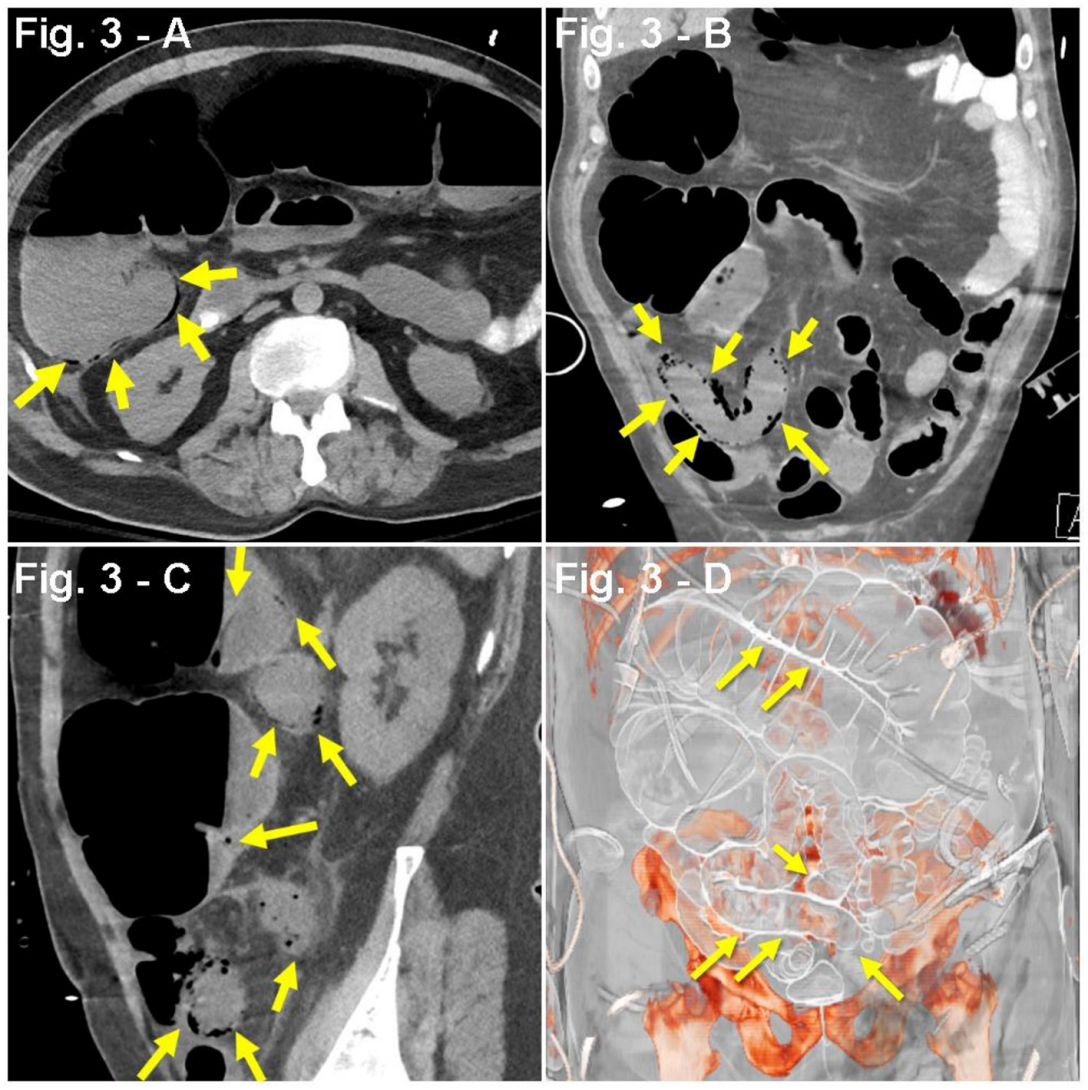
(A–D) Case presentation of a 63-year-old male patient, with dyspnea, cough, fever from COVID-19, with bloody diarrhea, and abdominal distention 3 days after receiving TCZ, abdominal CT with oral contrast only, (A) axial, (B) coronal, (C) sagittal, and (D) 3D reconstruction with consolidation seen along lung bases, and pneumatosis of small bowel loops (yellow arrows) with dilated small and large bowel loops consistent with ileus.
